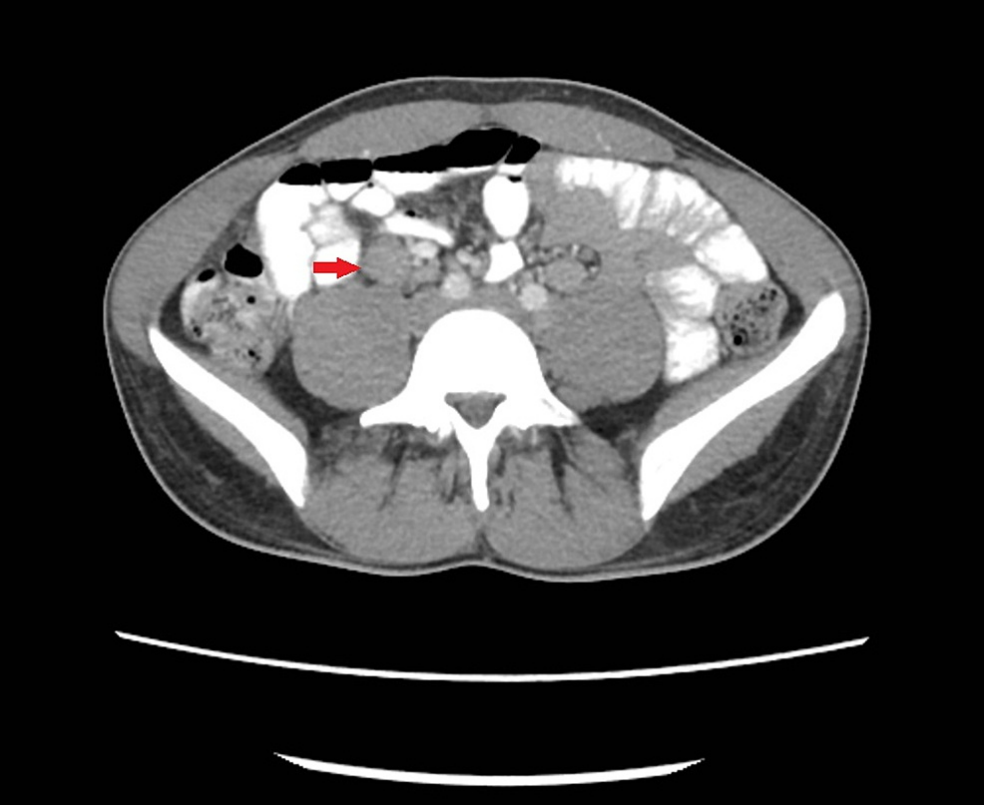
Axial image from CT of the abdomen and pelvis with oral and IV contrast demonstrates a cluster of enlarged mesenteric lymph nodes in the right lower quadrant (red arrow).
